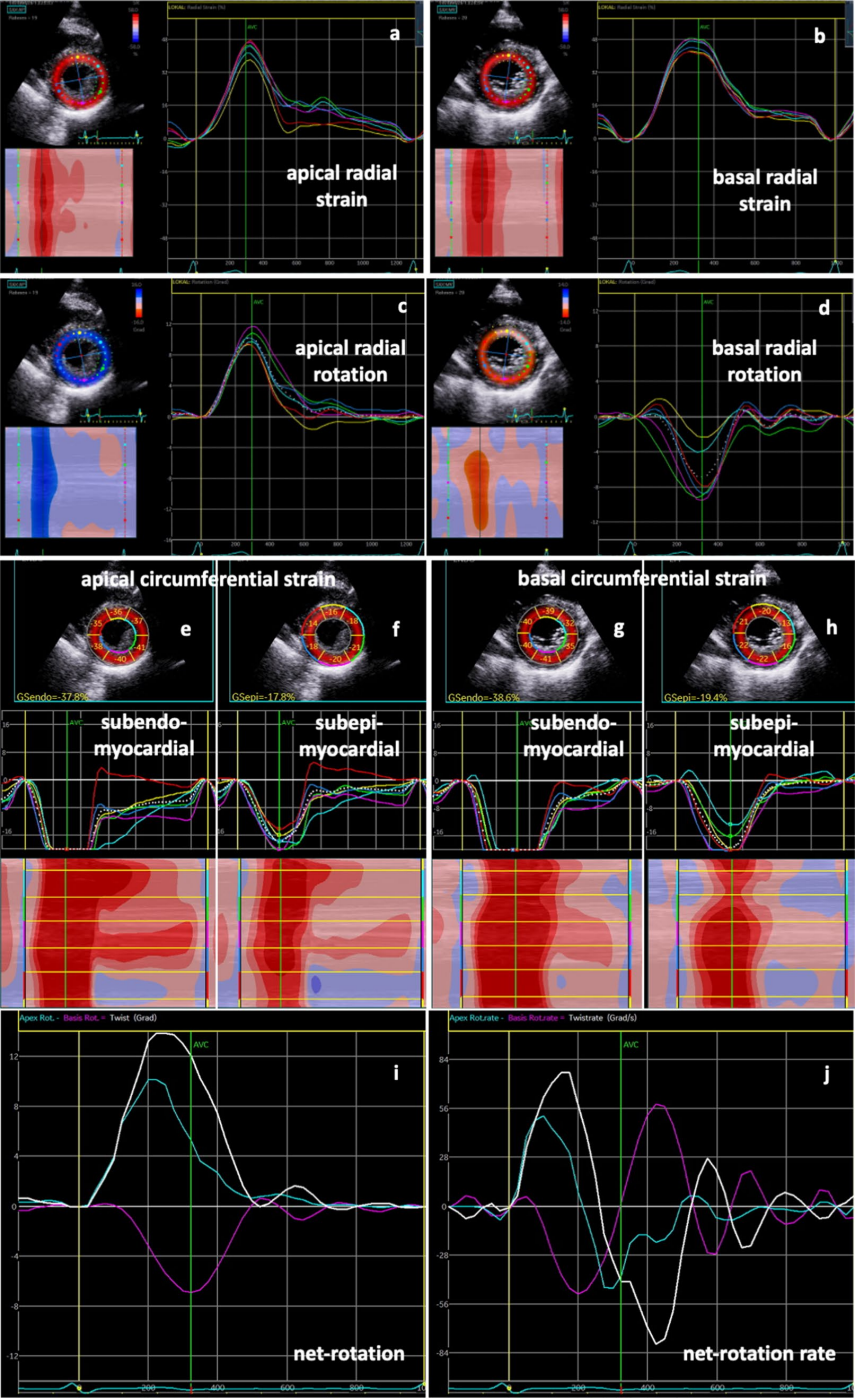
Rotational deformation pattern of a healthy individual: radial strain patterns in apical (a) and basal (b) left-ventricular (LV) segments as well as apical (c) and basal rotation (d) are documented. Below parasternal short-axis views with apical (e, f) and basal (g, h) segmental subendomyocardial (e, g) and subepimyocardial (f, h) circumferential strain values, strain curves, and color-M-Modes are shown. Line graphs of apical (blue) and basal rotation (magenta) as well as net rotation (twist) (white) (i) and corresponding line graphs of rotation rate (j) document normal findings
